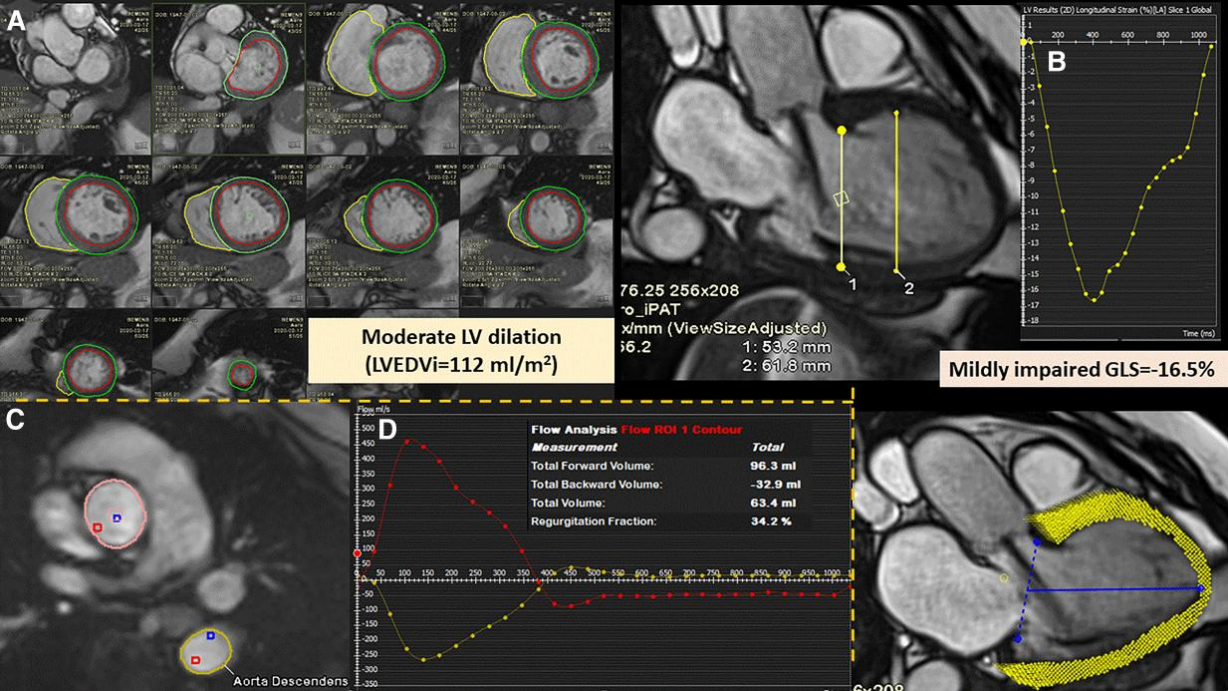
Magnetic resonance imaging for the assessment of aortic regurgitation severity and left ventricular remodelling (dilatation and dysfunction). A 72-year-old asymptomatic female patient with trileaflet aortic valve and echocardiogram showing moderate aortic regurgitation. (*A*) Breath-held short-axis stack cine acquisition for calculation of left ventricular volumes, ejection fraction, stroke volume, and left ventricular mass. There is moderate left ventricular dilatation (LVEDVi 112 mL/m^2^) despite a basal LVEDd measurement of 5.3 cm, vs. mid-left ventricular cavity (6.2 cm) correlates better with the volumetric mild left ventricular dilatation; left ventricular mass, wall thickness and LVEF (62%) are preserved. However in (*B*), global longitudinal strain is impaired = −16.5% (see the strain curve displayed in the upper panel and the myocardial overlay of the feature-tracking post-processing analysis in the lower panel). (*C*) 2D phase-contrast through-plane acquisition at the level of the ST junction for forward and backward flow and volume calculation. (*D*) Red curve indicates ascending aorta flow, whereas yellow curve, descending thoracic aorta. Severe aortic regurgitant fraction (34%) is identified. Holodiastolic retrograde flow in the descending thoracic aorta (yellow curve above the baseline) is a specific and supportive finding. Mild mitral regurgitation also identified (regurgitant volume 19 mL; regurgitant fraction 17%).
